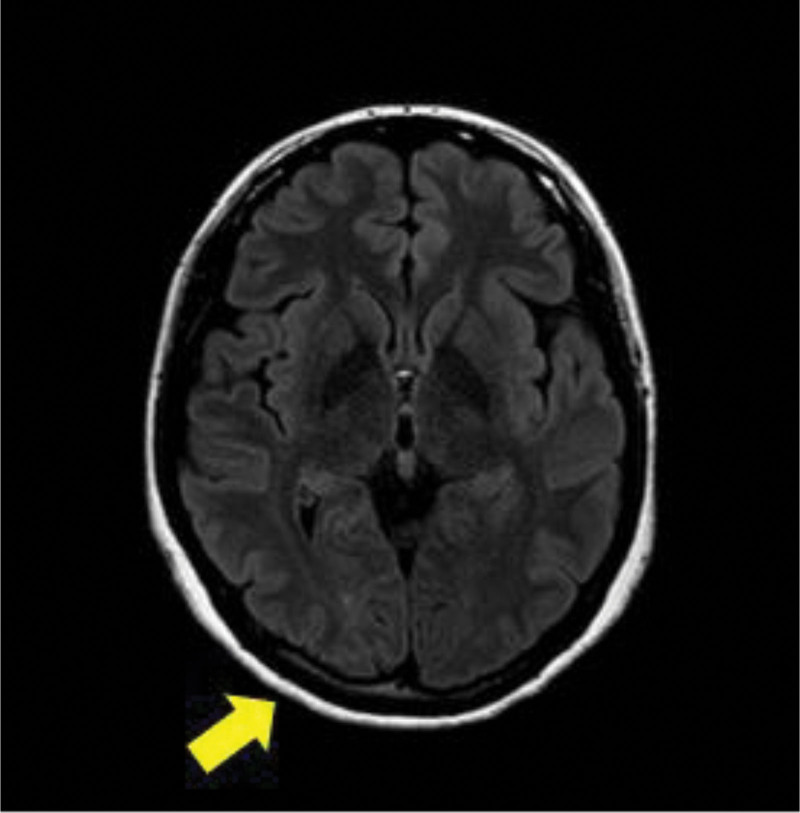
Brain MRI 3 months after onset. High signal intensity lesions in the parietal-temporal and occipital lesions disappeared in T2/FLAIR. MRI = magnetic resonance imaging.
